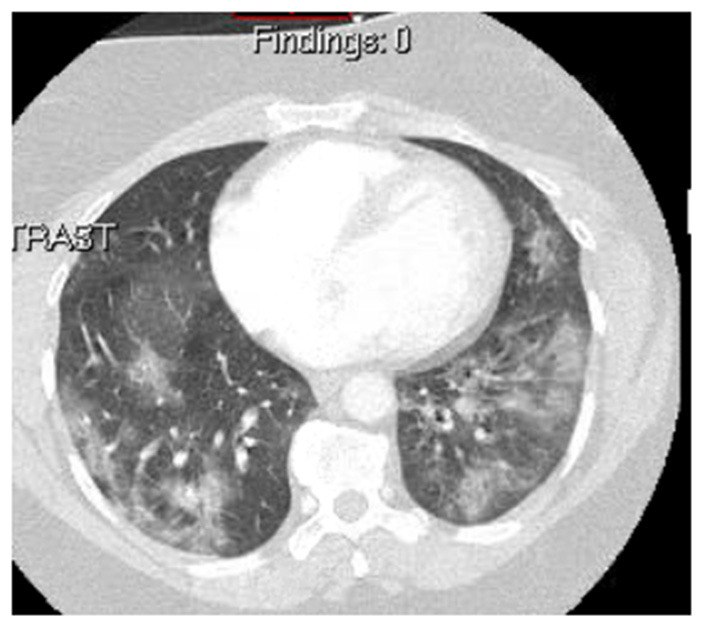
A chest CT of a 35-year-old woman with no significant past medical history presenting with progressive dyspnea, chest pain, and hemoptysis showing typical ground-glass opacities and consolidation, which is seen in COVID-19. The patient was confirmed of COVID-19 with PCR testing.
